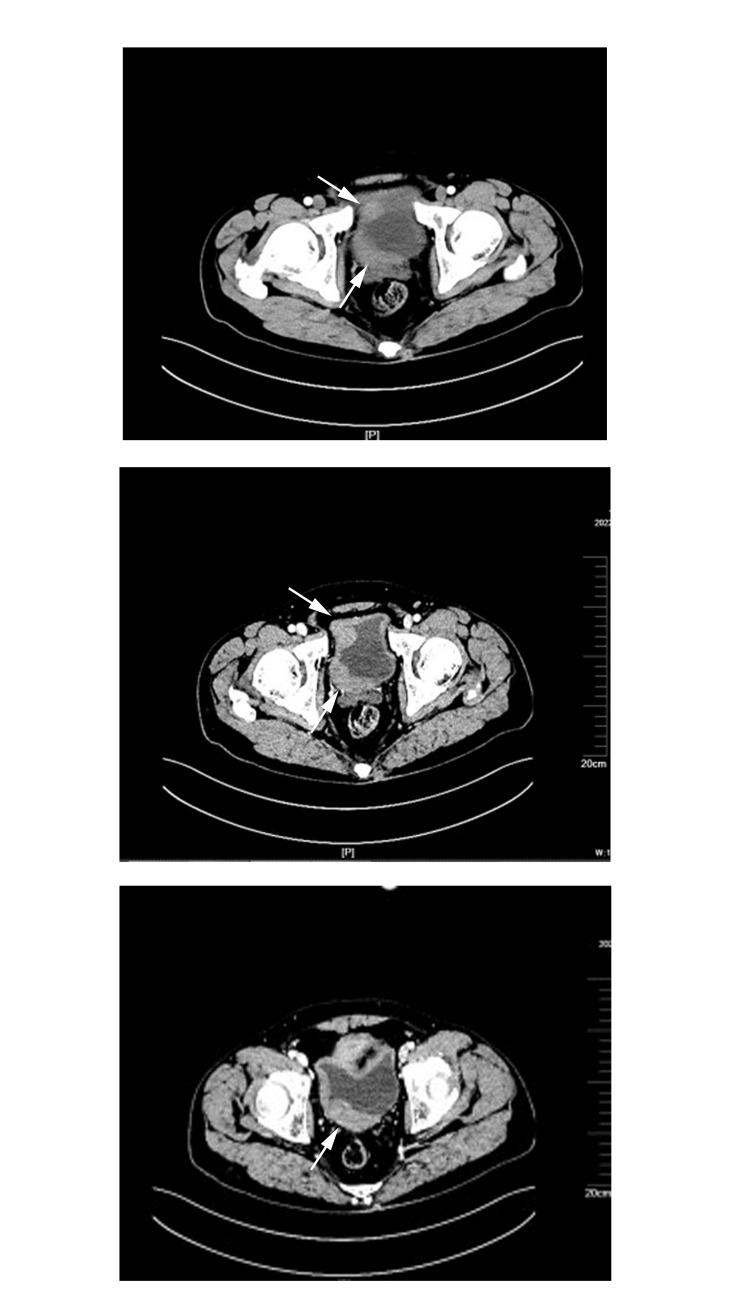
Representative CT of the bladder tumor The pelvic CT scan shows uneven thickening of the anterior wall, right wall, and posterior wall of the bladder (indicated by the arrows).
